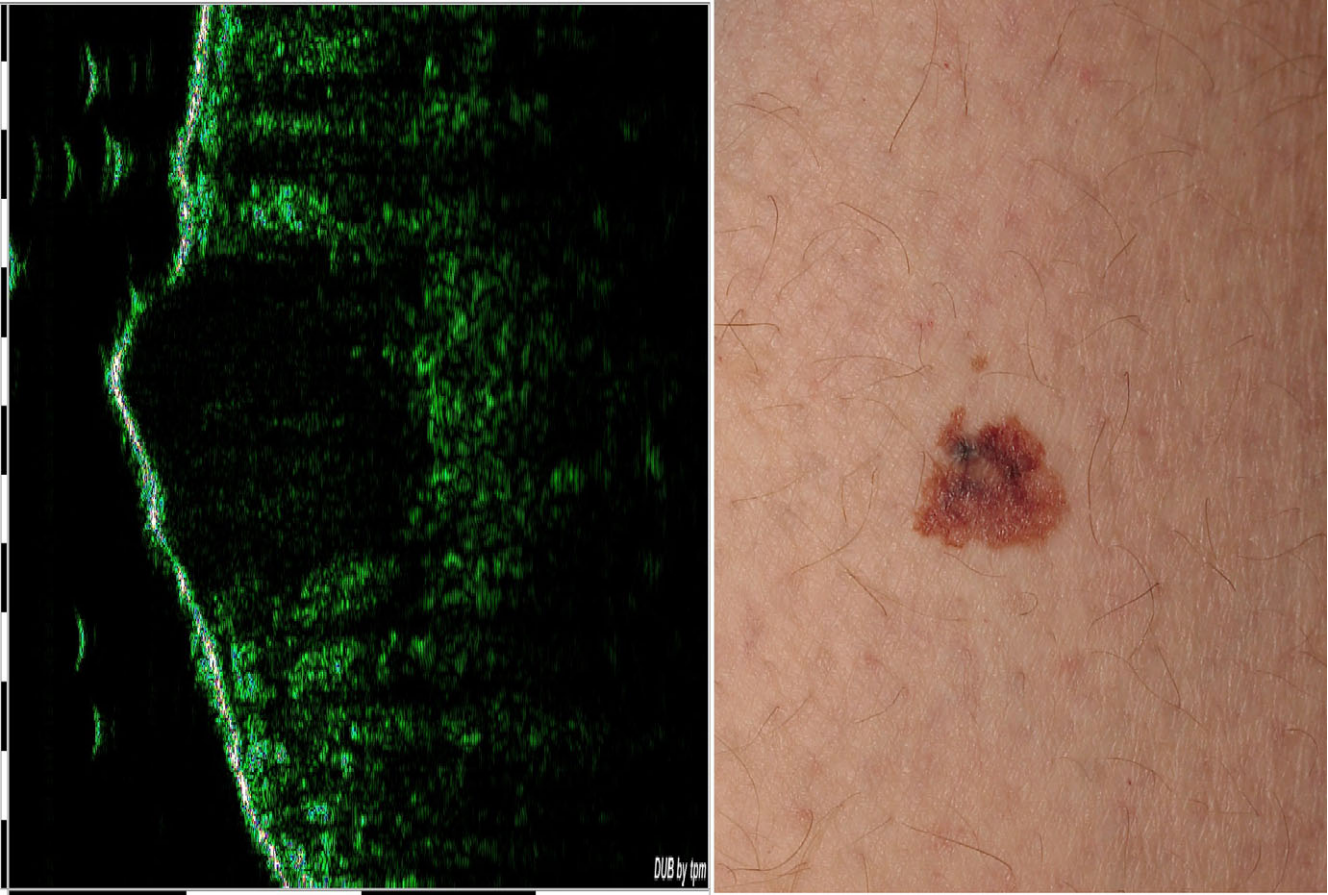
Sample of cancerous skin and its sonogram.
